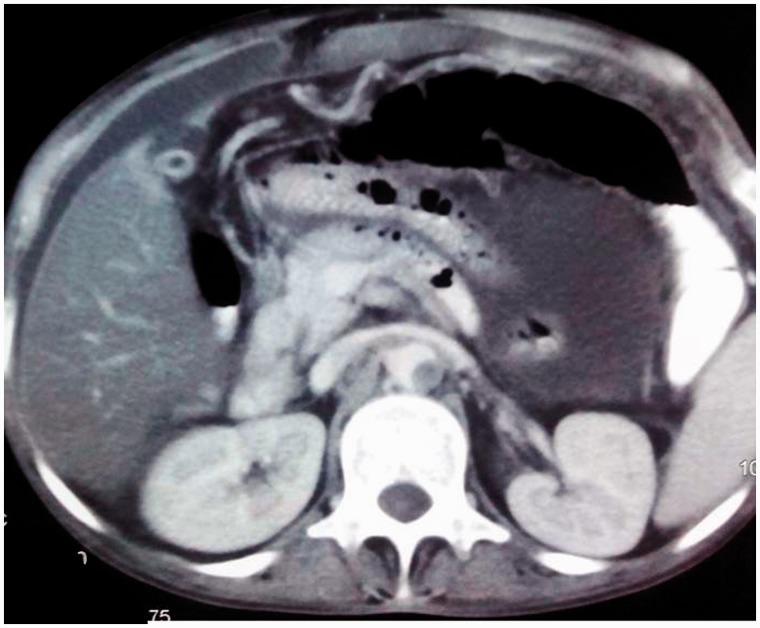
Computed tomography image of abdomen showing ascites, peritoneal enhancement and aortic thrombosis.
